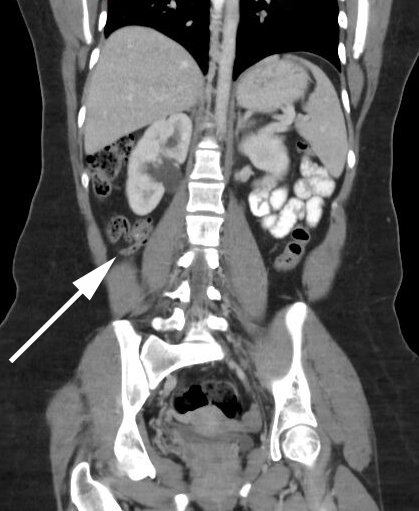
A 16-year-old female with abdominal mass (not pictured). A CT coronal image demonstrates a normal caliber appendix, coiled in the right mid abdomen. There is no evidence of mural thickening. The surrounding periappendiceal fat is pristine, and there is intraluminal gas. Incidentally noted is mild right sided urinary tract dilatation.
